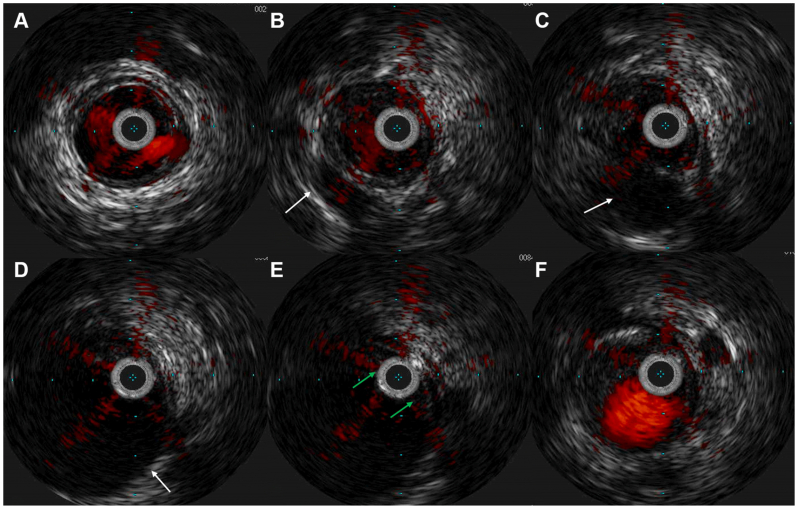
Intravascular ultrasound (IVUS) cross-sectional images of the popliteal artery from proximal to distal. (A) Proximal popliteal artery demonstrating the typical three-layered appearance of the arterial wall. (B-D) Progressive eccentric compression of the arterial lumen by a crescent-shaped cyst (*white arrows*). (E) Complete luminal compression caused by the cyst (*green arrows*). (F) Distal popliteal artery with preserved three-layered wall structure.
